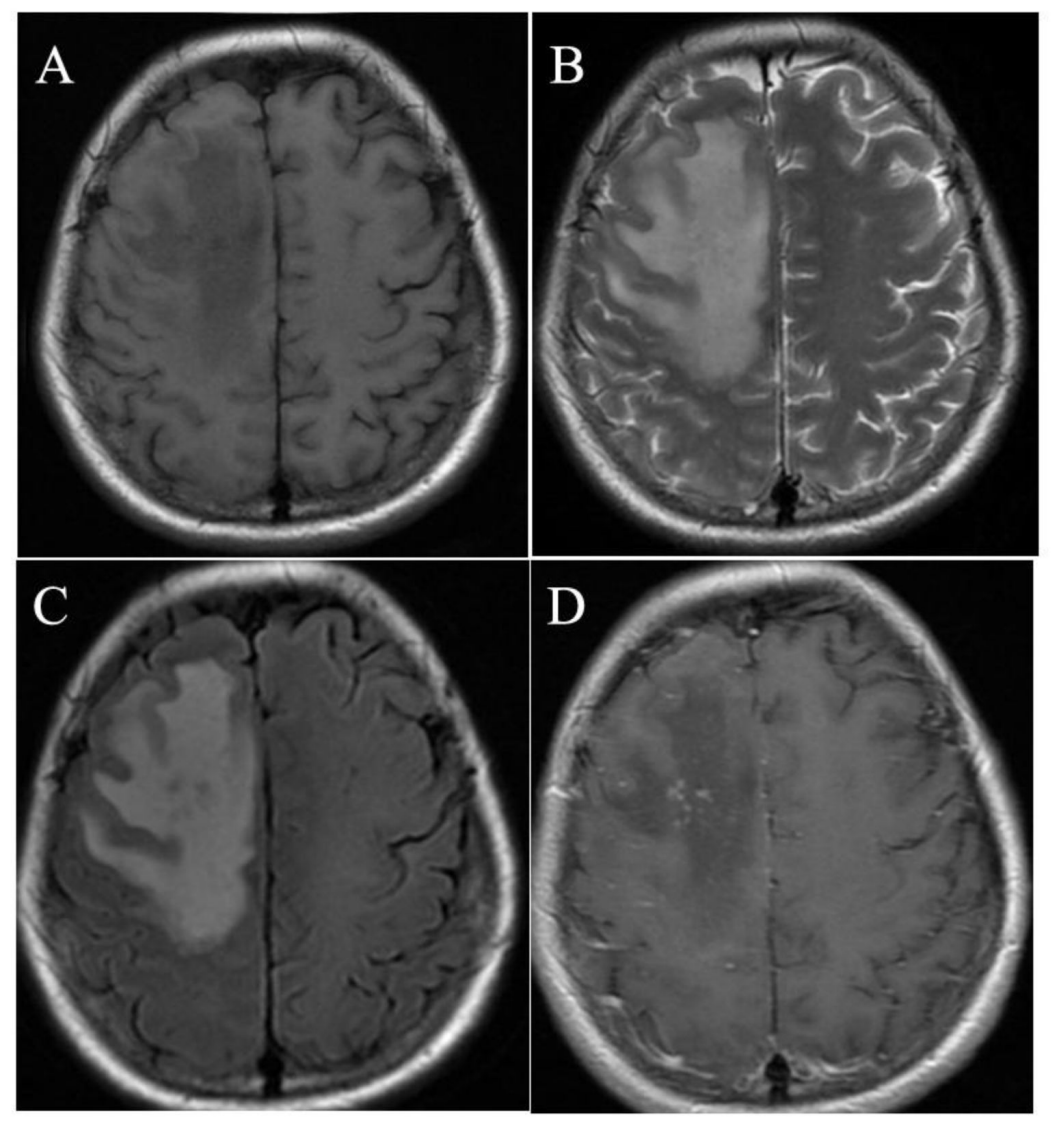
Brain magnetic resonance imaging of a 39-year old patient with encephalitis and acute flaccid paralysis due to SFTSV infection. A. Axial T_1_-weighted image shows hypointense lesion in the right frontoparietal lobes. B. Axial T_2_-weighted image shows the frontoparietal lesion is hyperintense with gyral swelling. C. Axial Flair sequence image shows the lesion is hyperintense with some isointense dots inside. D. Contrast-enhanced T_1_-weighted image shows marked dot-like enhancement in the lesion.
